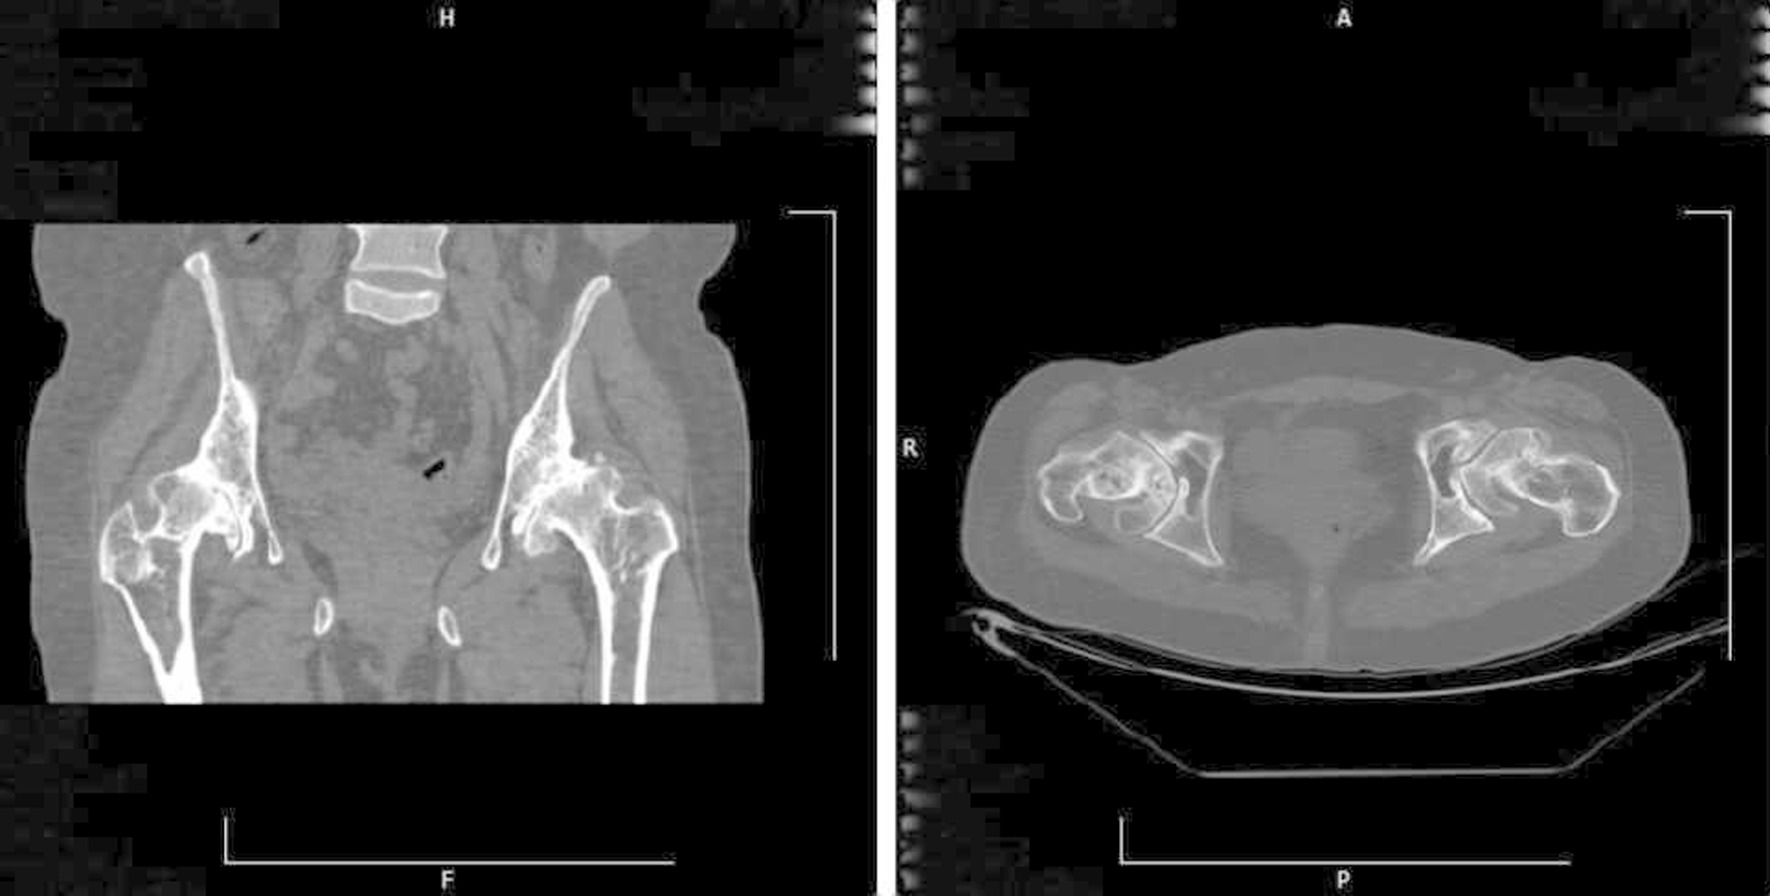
The pelvic Spiral CT scan in coronal (right) and axial (left) views
